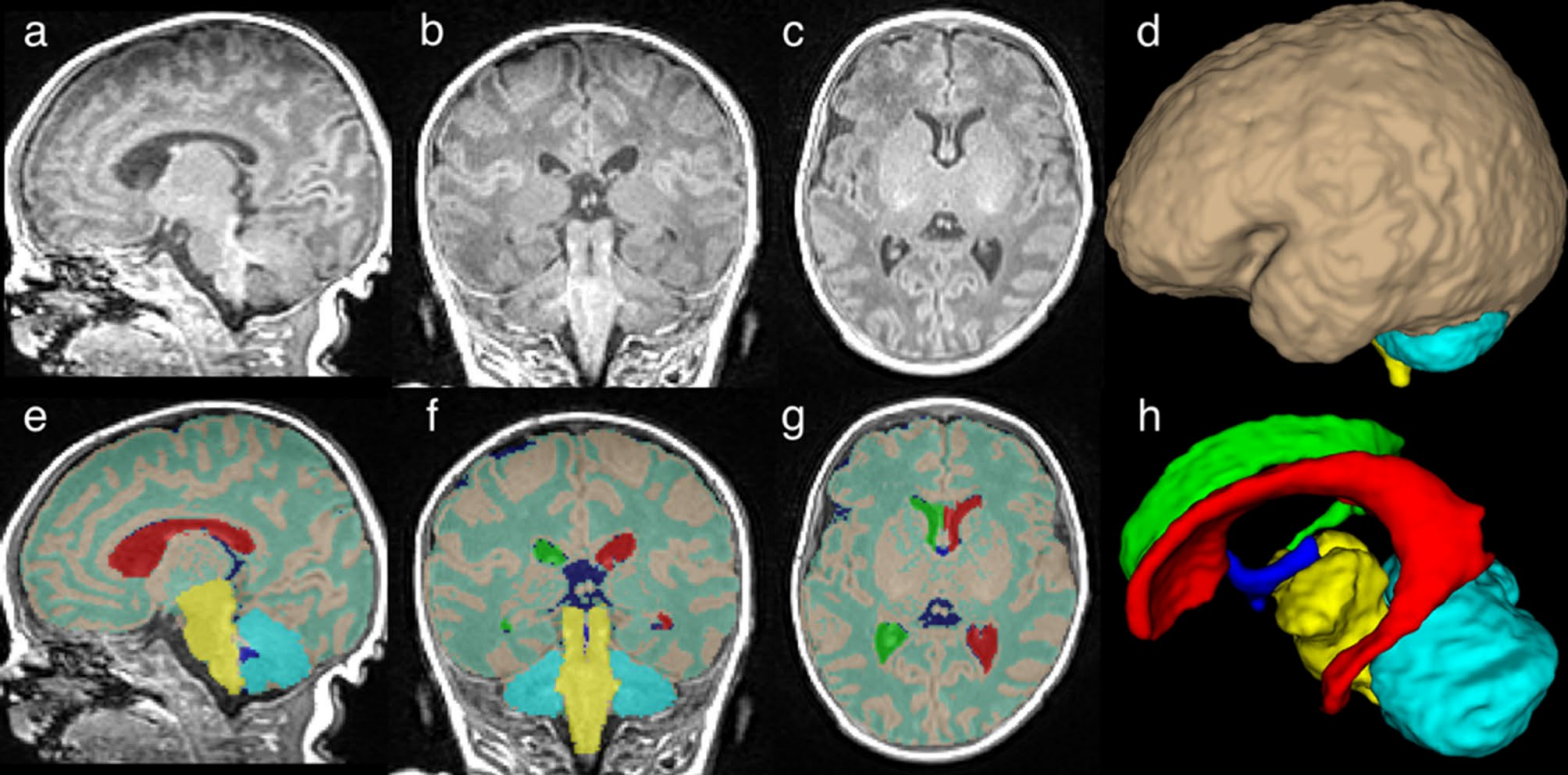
Manually segmented anatomical regions overlaid on a T1-weighted image. T1-weighted image with no overlay in sagittal (a), coronal (b), and axial (c) slices through the volume. Manually segmented anatomical regions overlaid on the same T1-weighted image in sagittal (e), coronal (f), and axial (g) slices through the volume. 3D renderings of the segmentations are shown in (d) and (h). Red label: left lateral ventricle. Green label: right lateral ventricle. Dark blue: 3rd and 4th ventricles. Yellow: brain stem. Cyan: cerebellum. Turquoise: white matter. Brown: grey matter.
